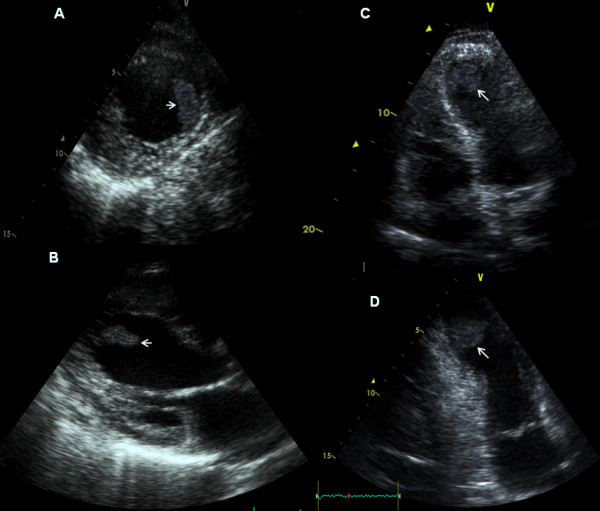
Transthoracic echocardiography of the left ventricle. Thrombus (arrows) in the apex of the left ventricle is clearly depicted in the (A) short, (B) long parasternal axes as well as in the (C) apical four-chamber and in the (D) apical two-chamber views.
